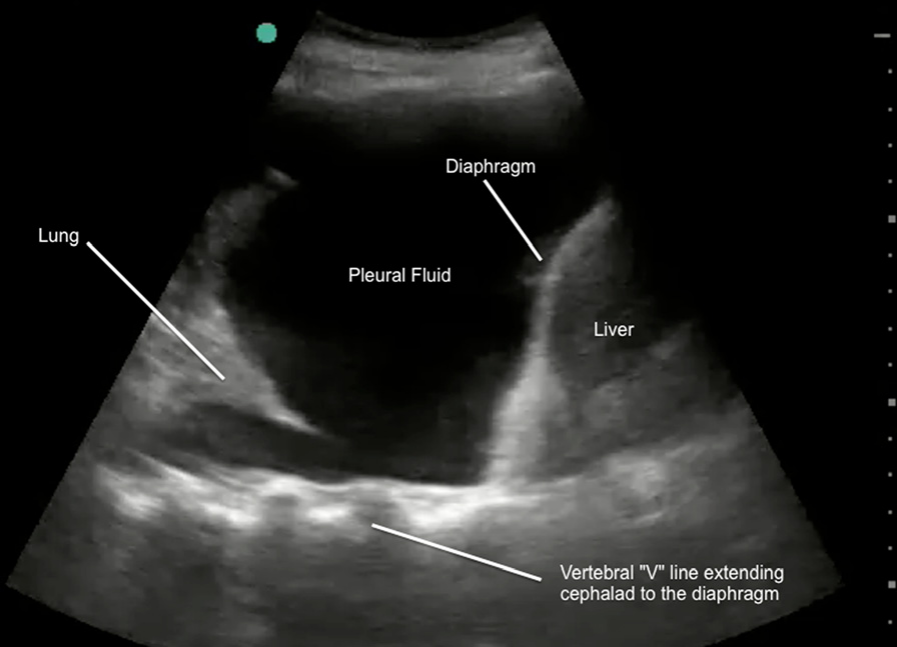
Lung ultrasound demonstrating V line (echogenic posterior line extending above the diaphragm). This confirms pleural fluid (right lung base).
